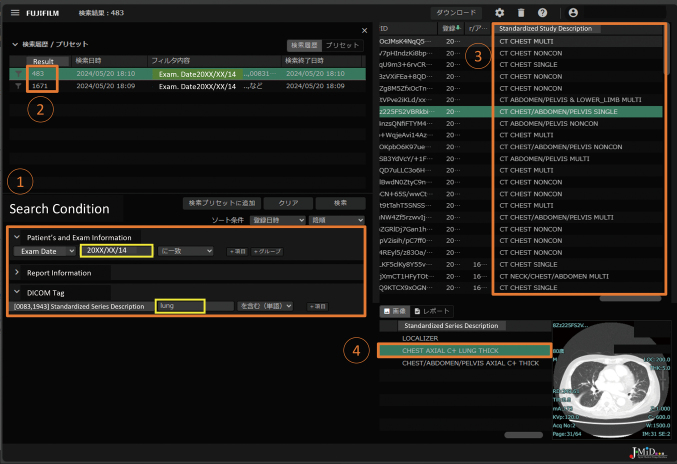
Study list of J-MID This was the search screen for the J-MID study list. 1,2) 1,671 studies were conducted at ‘20XX/XX/14’ in 10 hospitals. Among them, 483 series included ‘lung’ in ‘Standardized Series Description’. 3) A result list includes ‘CHEST’ in the ‘Standardized Study Description’ as a body part of the imaging. 4) A series of lung window images with the standardized series description of ‘CHEST AXIAL C+ LUNG THICK’ is displayed in a study of ‘CT CHEST/ABDOMEN/PELVIS SINGLE.’
